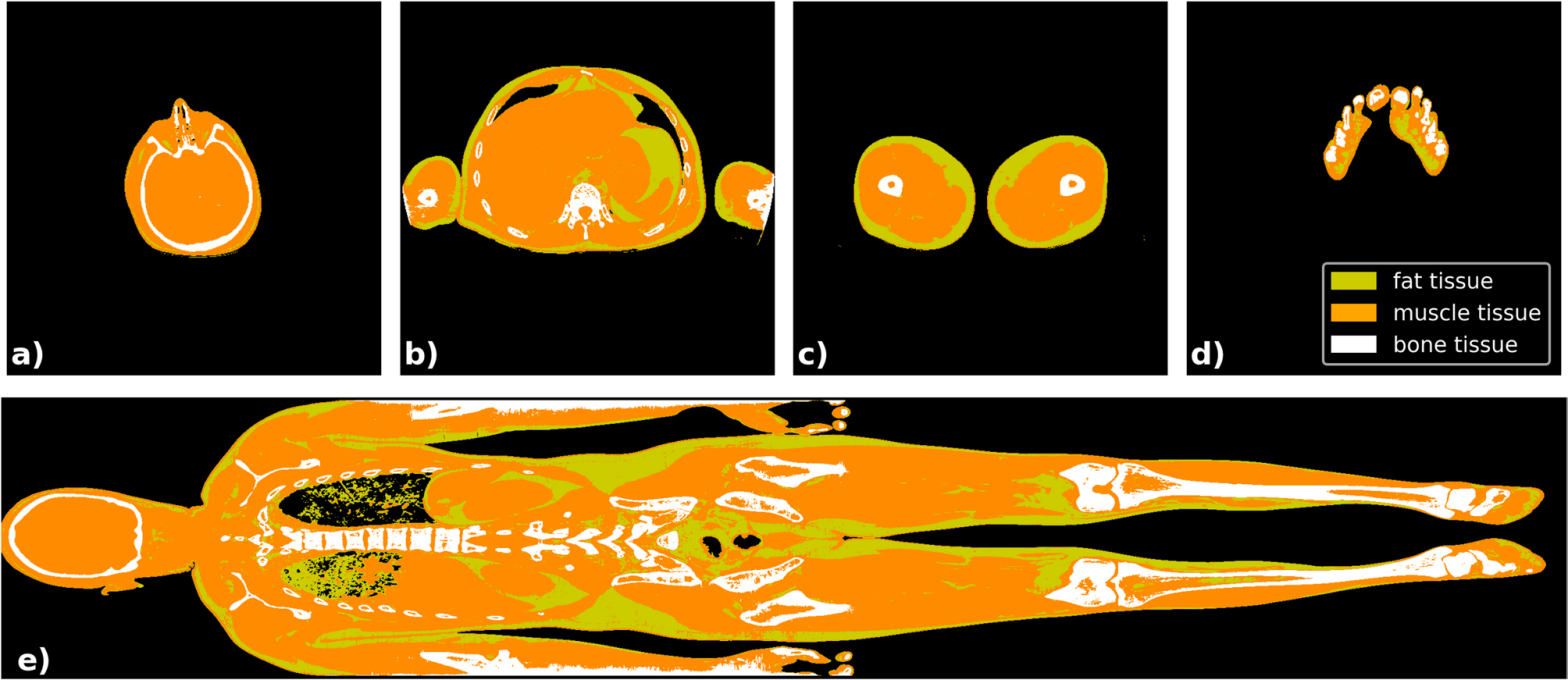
An exemplary visualization of the differentiation between fat, muscle, and bone tissue through thresholding, shown in transverse (a–d) and coronal (e) slices of the whole-body postmortem CT images. If the field of view of the CT scanner is insufficient, sections such as the arms may be partially cut off in the CT images.
